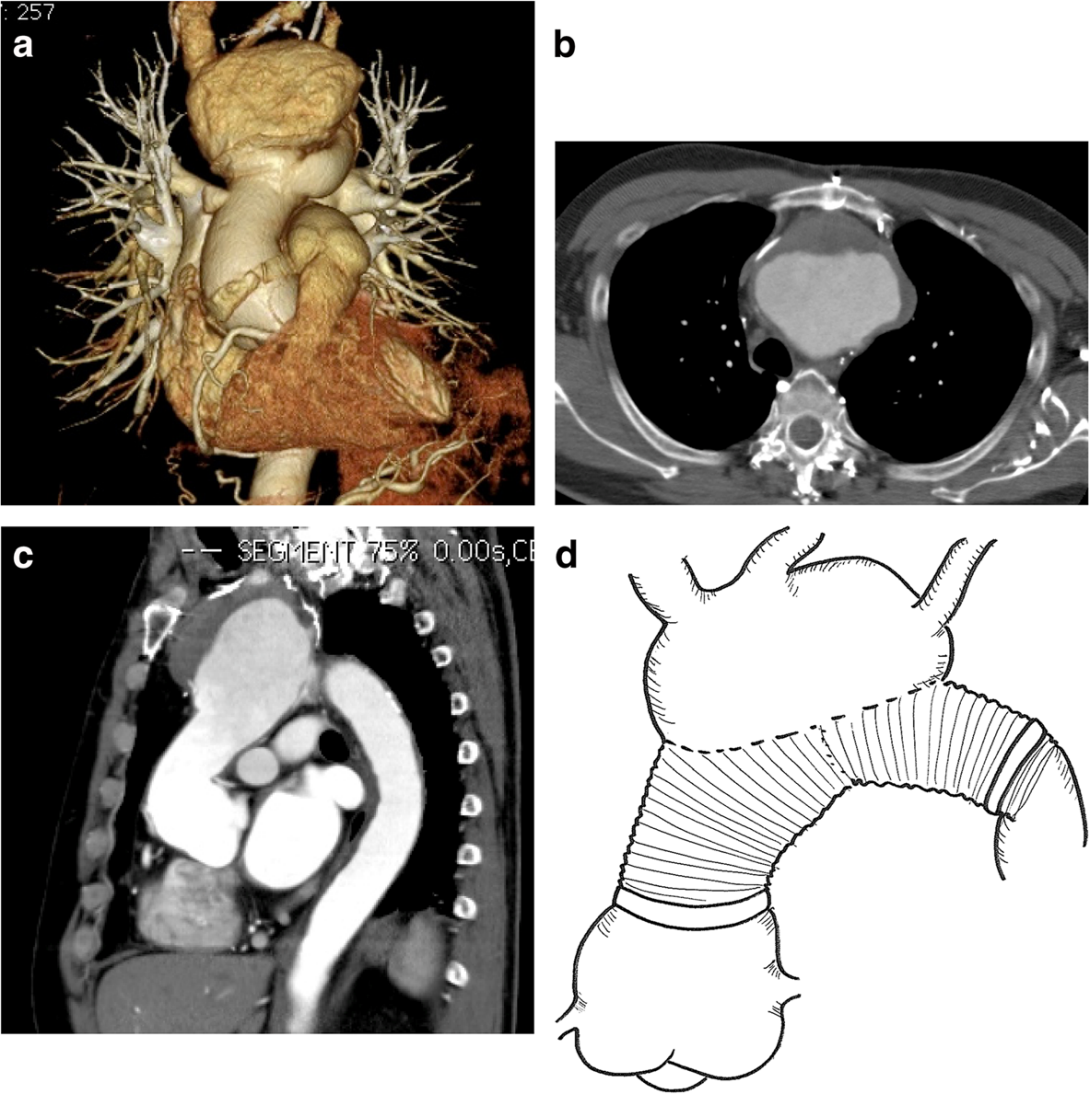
Multidetector computed tomography (MDCT) 11 years after the previous surgery. The MDCT demonstrates a giant arch anastomotic pseudoaneurysm, aortic root enlargement and coronary artery stenosis, and shows no re-dissection in the pseudoaneurysm. a Synthetic image, (b-c) axial view, and (d) scheme of the aneurysm
